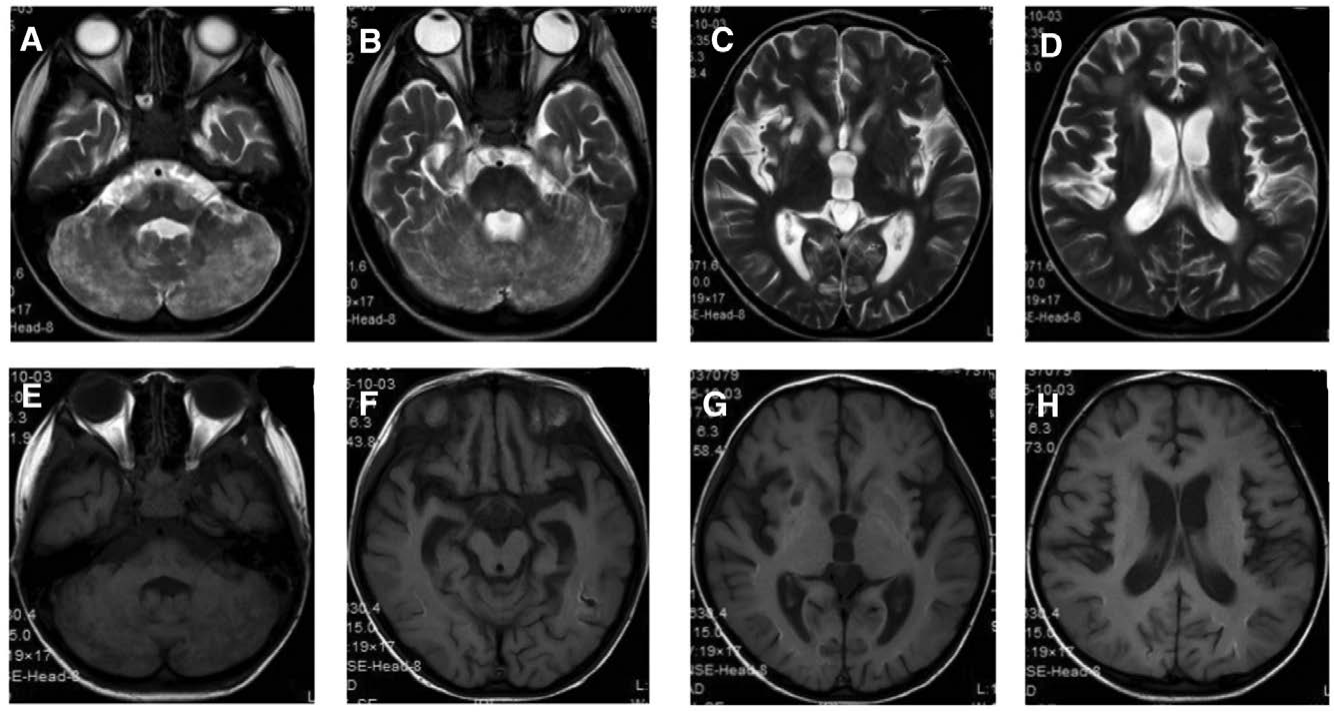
Cranial MRI of Case 2 at the age of 5 years and 9 months. Multiple sporadic intracranial abnormal signals accompanied by layer necrosis of the cortex. Also, the formation of partial encephalomalacia was seen. MRI = magnetic resonance imaging.
